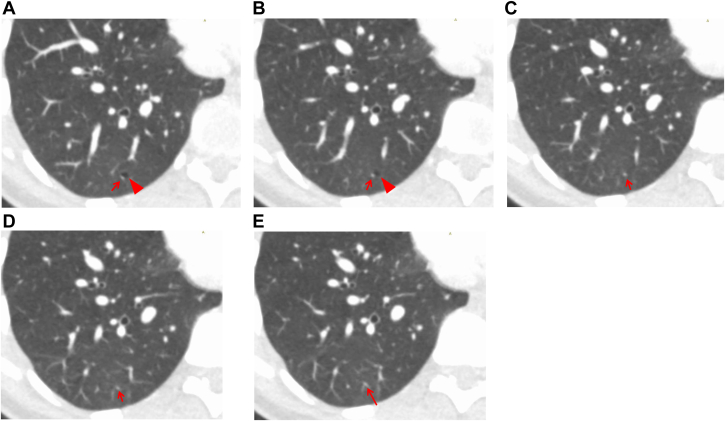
A-E, Successive premenstrual CT scans from the caudal to the cranial direction. Successive CT images lie from the caudal (A) to the cranial (E) direction. Arrows indicate pulmonary artery, and arrowheads indicate dilated bronchus.
